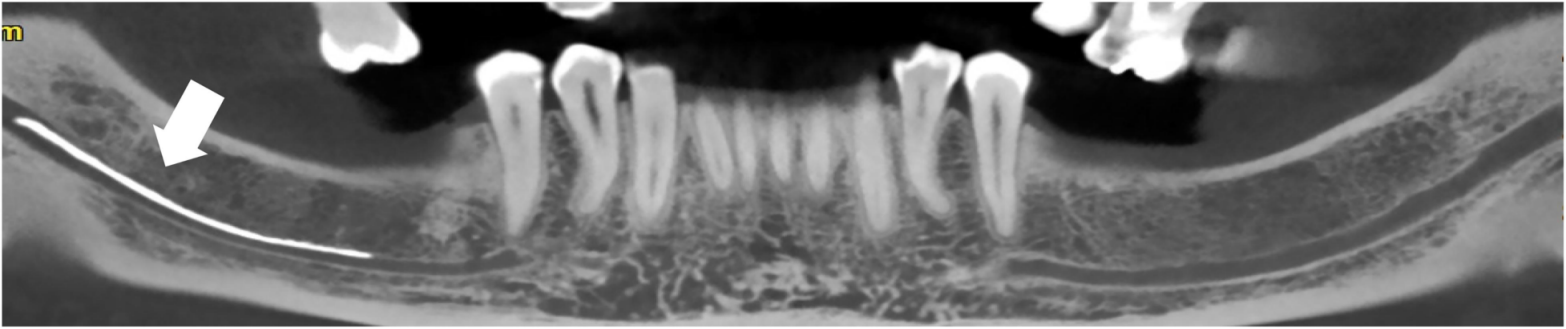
Coronal CBCT section taken after 2 years of follow-up revealed bone healing following extraction of the tooth 46, with persistence of the material in the IAN canal and slight resorption.
